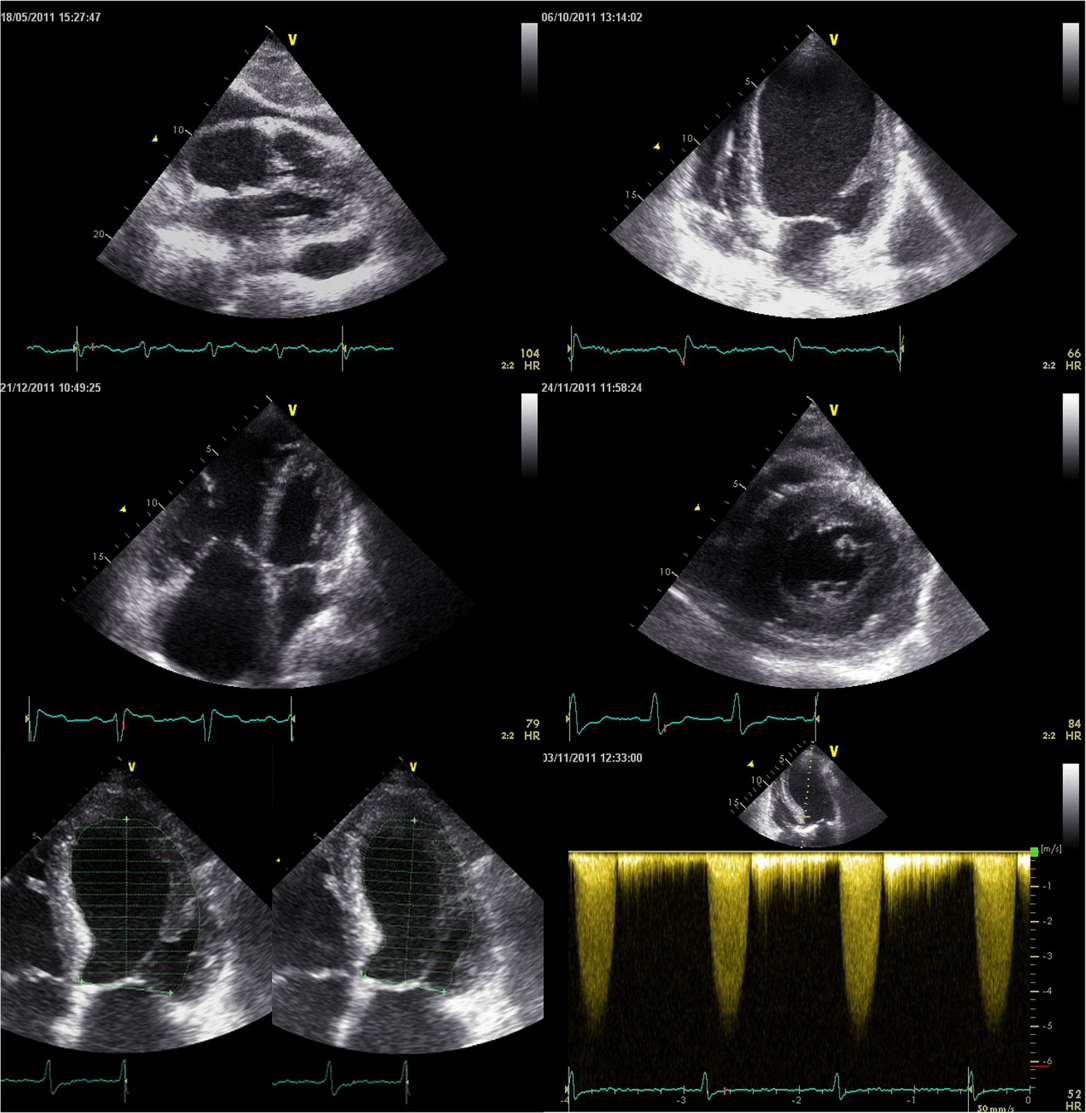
Examples of severe pathology detected by POC ultrasonography at the bedside. Images were obtained by the novice examiner. *Upper left panel:* Pericaldial exudates. *Upper right panel:* Left ventricular dilation. *Middle left panel:* Right ventricular dilation. *Middle right:* Myocardial hypertrophy. *Lower left:* Impaired ejection fraction. *Lower right:* Aortic stenosis.
